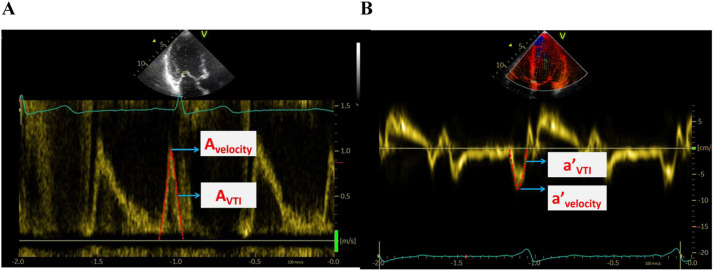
Late transmitral flow A-wave measurement by pulse Doppler (A), and late diastolic a’-wave of the septal/lateral mitral annulus measurement by tissue Doppler imaging (B). A_velocity_, Peak Velocities of transmitral flow A-wave; A_VTI_, Velocity-Time integral of transmitral flow A-wave; a’_velocity_, Peak velocities of septal/lateral mitral annulus a’-wave; a’_VTI_, Velocity-time integral of septal/lateral mitral annulus a’-wave.
